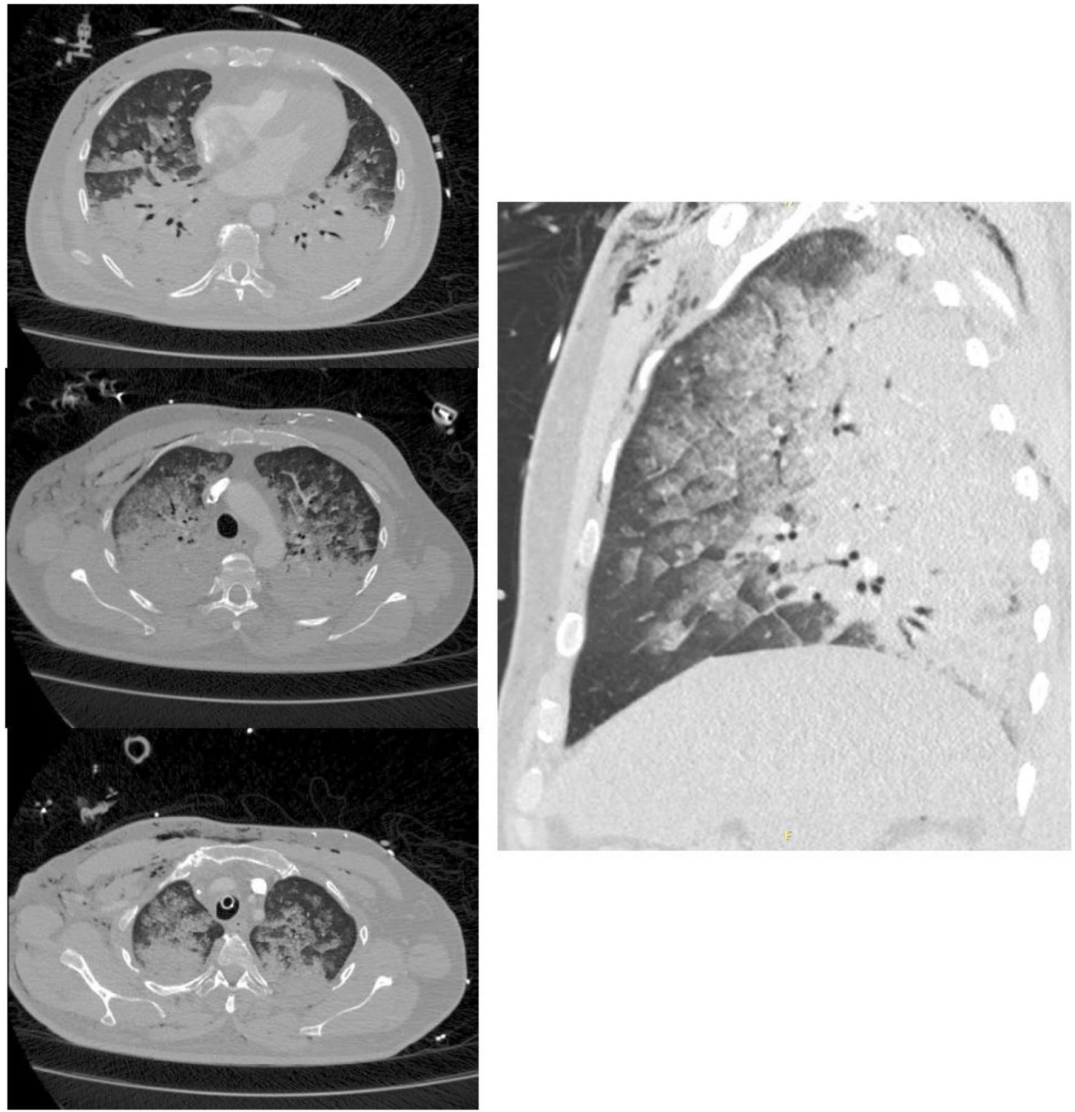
CT-scan with angiography of the thorax. Approximately 20 min post-intubution on postoperative day 0. Three transversal views from supradiaphragmal to apical (left). One sagittal view (right). Presence of severe alveolar interstitial bi-pulmonary edema. No signs of pulmonary artery embolism. Postoperative ventral subcutaneous emphysema
